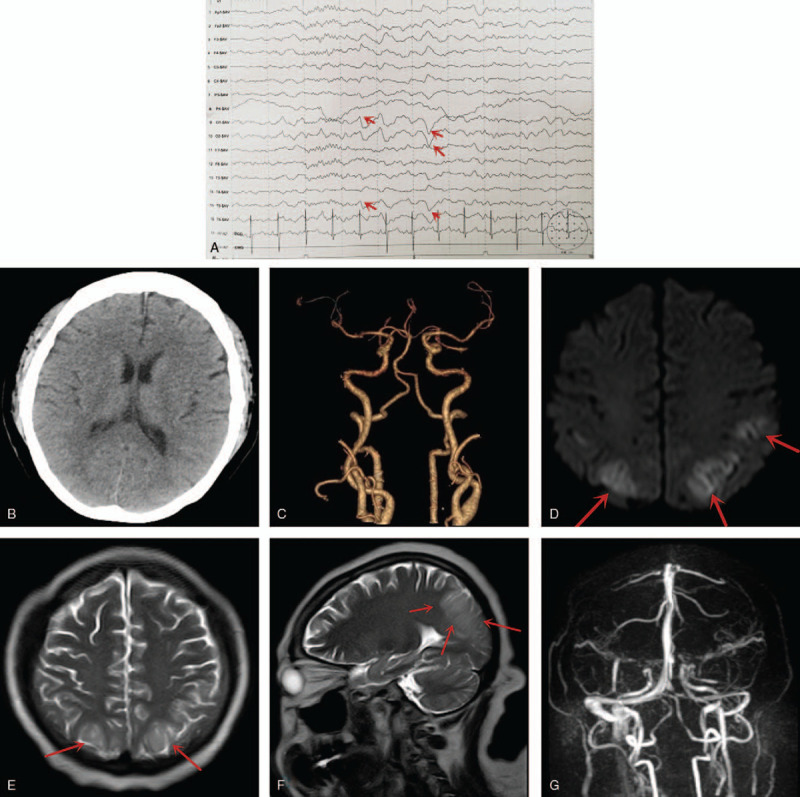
(A) Is an EEG showing abnormal occipital lobe apical waves consistent with acute seizure disorder. Red arrows = abnormal waves. (B and C) Are head CT (B) scan and CTA (C) showing no abnormalities. (D–F) Are MRIs showing abnormal signals at both temporo-parieto-occipital cortices, the subcortical area, as well as the left hippocampus. (G) Is an MRA showing no vascular abnormality. CT = computer tomography, CTA = CT angiography, MRA = magnetic resonance angiography.
